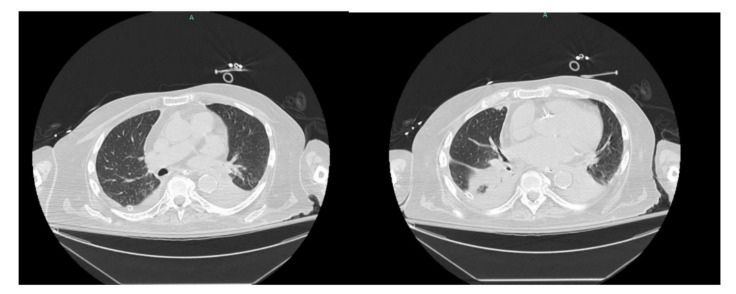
CT chest showing diffuse narrowing of the left mainstem bronchus with trace right pleural effusion.
